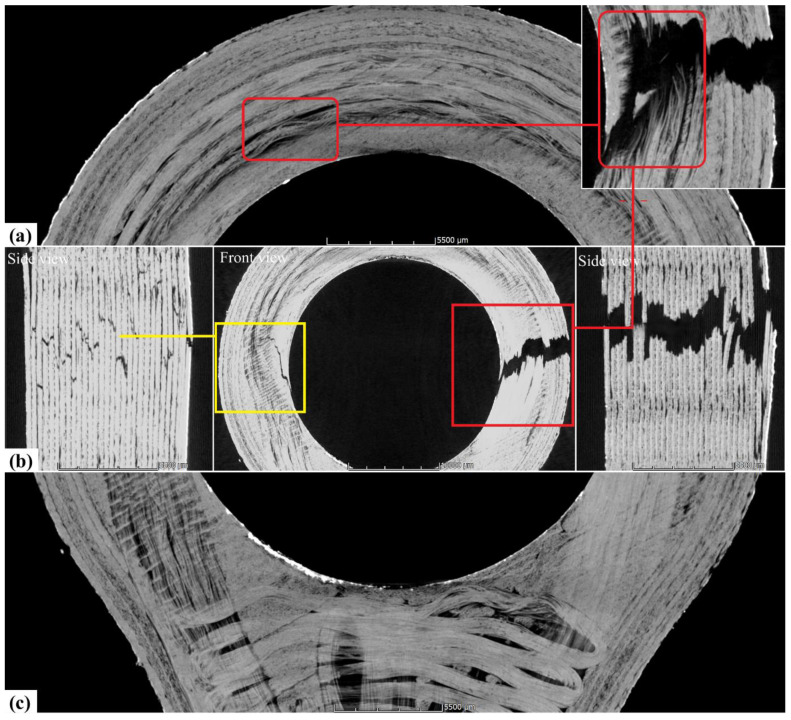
CT scan of the fractured lug with an overview of two fractured zones. Maximum principal stress direction CCF layup with a primary fractured zone as an inset (a); overview and side views of primary (red frame) and secondary (yellow frame) fractures (b); minimum principal stress direction CCF layup (c). The red connecting line indicates the cause and effect in the fractured region (undulation and voids).
